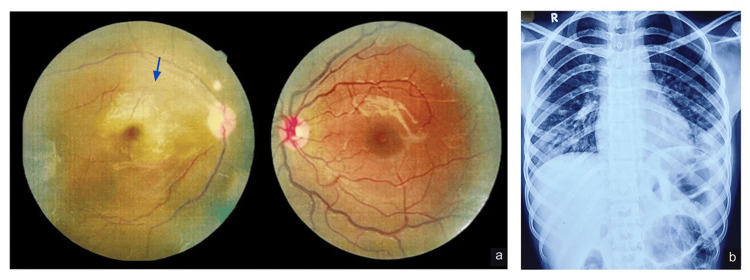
Imaging results performed at the referring centre (a) Fundus photograph done one month before presentation to our hospital suggestive of OD acute presentation of Branch Retinal Artery Occlusion (BRAO) involving macula with Cherry Red Spot (blue arrow), OS vascular tortuosity. (b) X-ray Chest showing small patches of fluffy radio opacities in bilateral lung fields sparing upper lung fields suggestive of small air space consolidation/pneumonitis, likely due to infective etiology. OD - Right eye, OS - Left eye.
